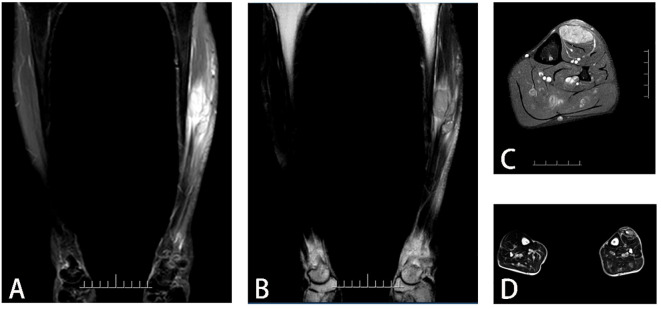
Results of gastroscopy, endoscopic ultrasound, and abdominal contrast-enhanced CT two months after left thigh amputation. (A, B) Gastroscopic views revealing metastatic rhabdomyosarcoma in the stomach, showing ulcerative and nodular mucosal lesions. (C, D) Endoscopic ultrasound images demonstrating hypoechoic masses consistent with gastric metastasis from rhabdomyosarcoma. (E, F) Axial contrast-enhanced CT scans of the abdomen/pelvis showing small bowel obstruction due to metastatic rhabdomyosarcoma, with dilated loops and wall thickening.
